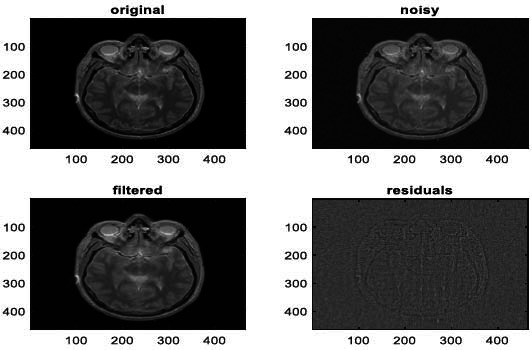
The fifth example of MRI denoising by applying the proposed image denoising technique.
